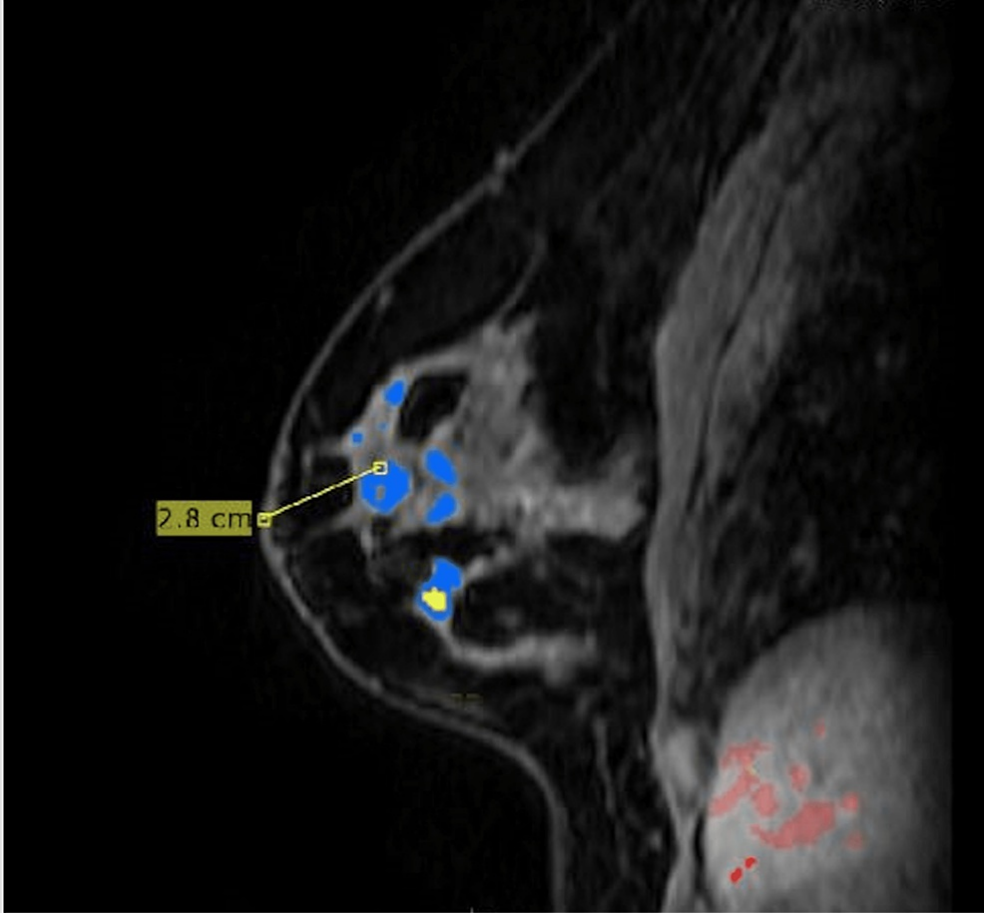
Additional mass found at 11 o'clock MRI findings stated that "At the 11 o'clock position, approximately 2.8 cm from the nipple, there are two adjacent masses, measuring 2.0 in diameter."
